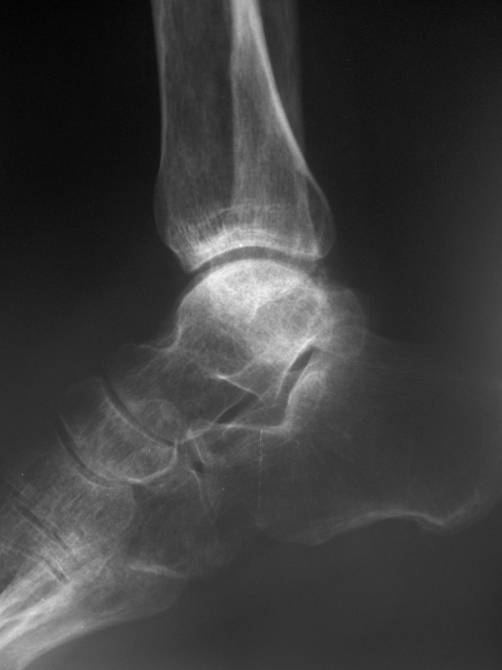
Lateral radiograph of the ankle 3 years post-injury. Sclerosis of the body of the talus and degenerative changes in ankle and subtalar joints are evident.
